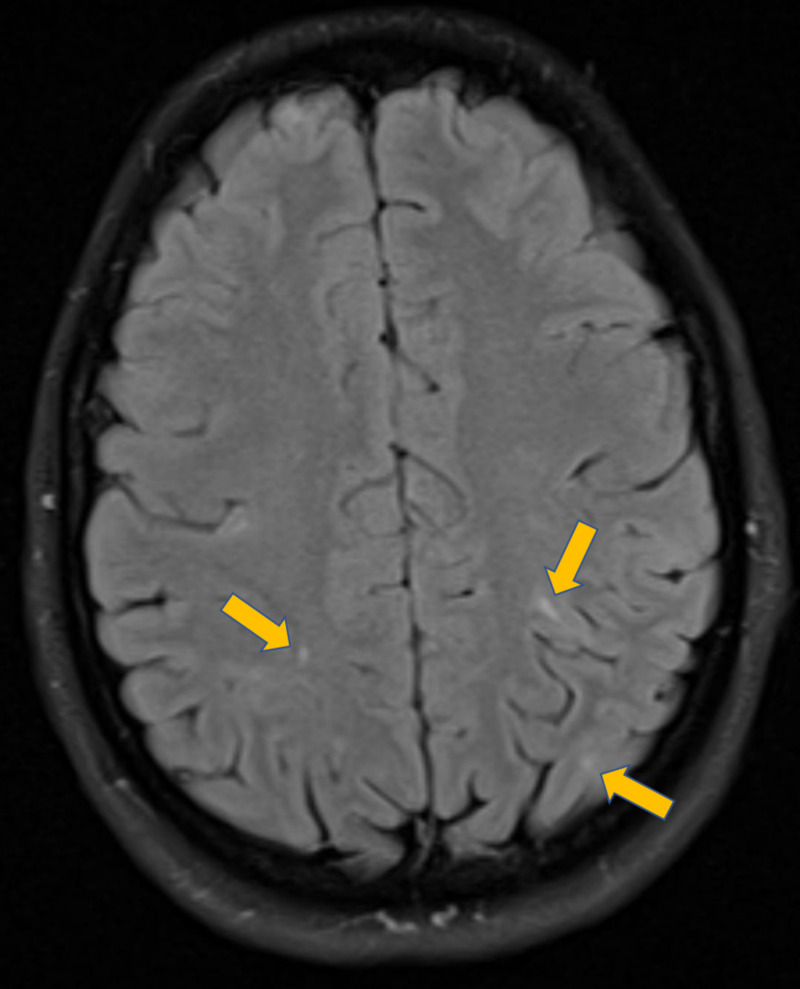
MRI brain showing multiple tuberii MRI: magnetic resonance imaging.
